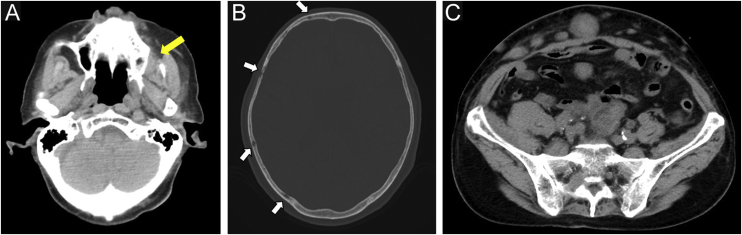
Computed tomography findings. (A) Soft tissue tumor in the left maxillary sinus (yellow arrow). (B) Osteolytic changes in the skull (white arrows). (C) Multiple abdominal subcutaneous and intraperitoneal metastases.
